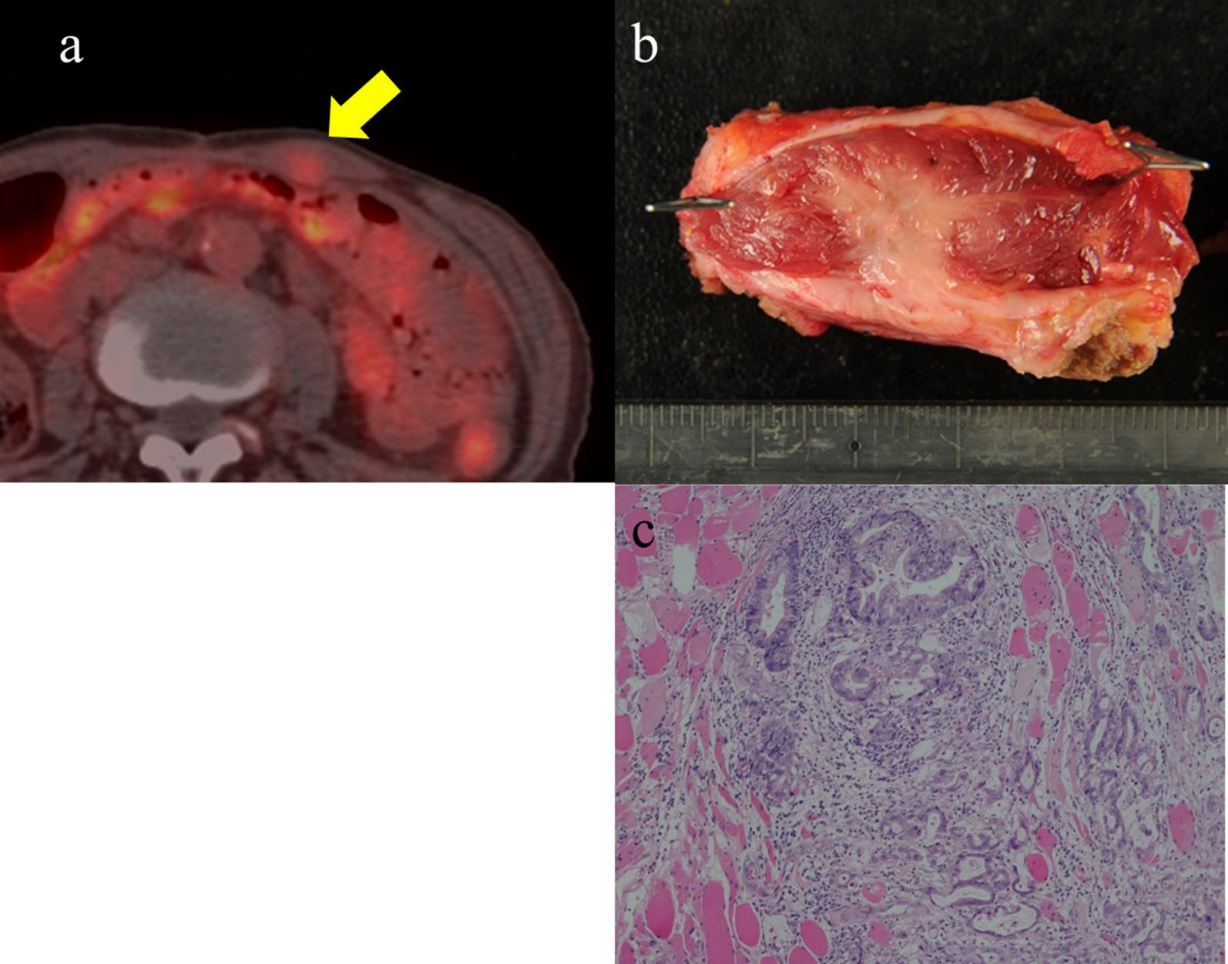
Computed tomography (CT) scan and pathological findings of the second recurrence. a Positron emission tomography (PET)/CT scan showing an elevated fluorodeoxyglucose (FDG) intake in the left rectus abdominis muscle. b Macroscopic findings showing a white nodular lesion in the skeletal muscle. c Pathological examination revealing an adenocarcinoma consistent with metastatic tumor from gastric cancer
